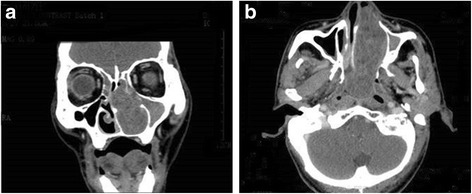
a A coronal CT image showing a soft tissue mass filling the left nasal cavity, causing obstruction of the left frontal and ethmoid sinuses. b Axial enhanced CT showed a soft tissue mass in the left nasal cavity featuring mild to moderate heterogeneous enhancement. The tumor extends posteriorly to the choana and nasopharyngeal region, with an indistinct border from the soft tissue of the lateral nasopharyngeal wall
